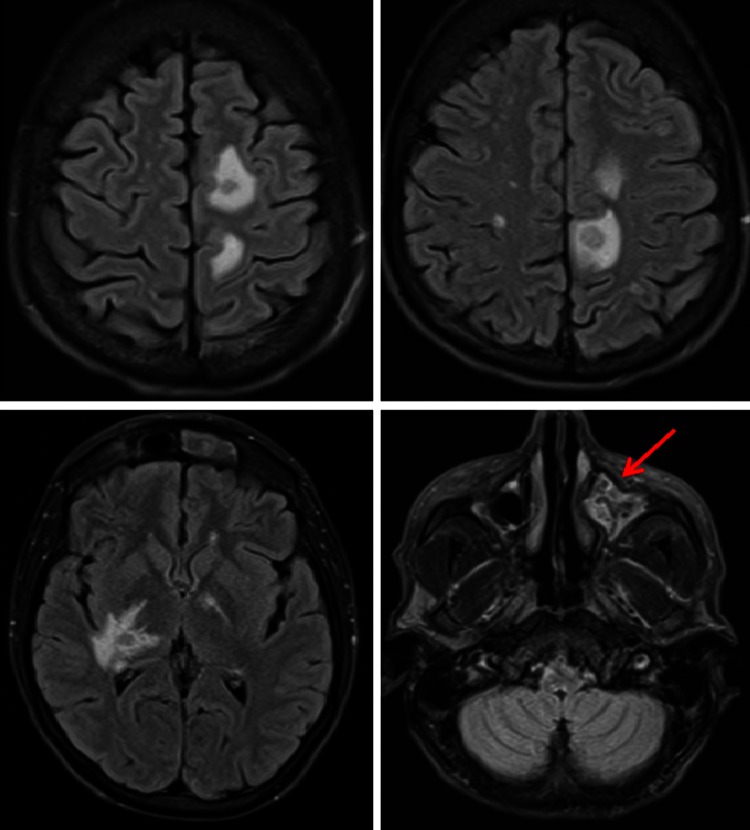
MRI scan of the brain in a patient with acute myeloid leukemia and CNS aspergillosis. It shows multiple ring enhancing lesions in the internal border zone bilaterally (border zone between lenticulostriate perforators and the deep penetrating cortical branches of the middle cerebral artery (MCA) or at the border zone of deep white matter branches of the MCA and the anterior cerebral artery. *Red arrow* shows thickening of the mucosa of the frontal sinus due to acute sinusitis
